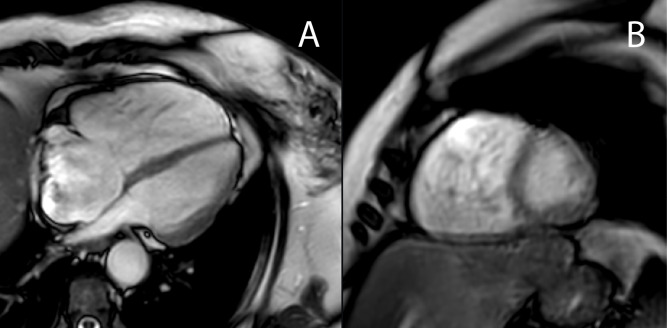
Right heart dilation and diastolic ‘D‑shaping’: axial (A) and sagittal (B) cine cardiac MR images showing dilation of the right atrium and ventricle with flattening of the interventricular septum ‘D‑shaping’ during diastole.
